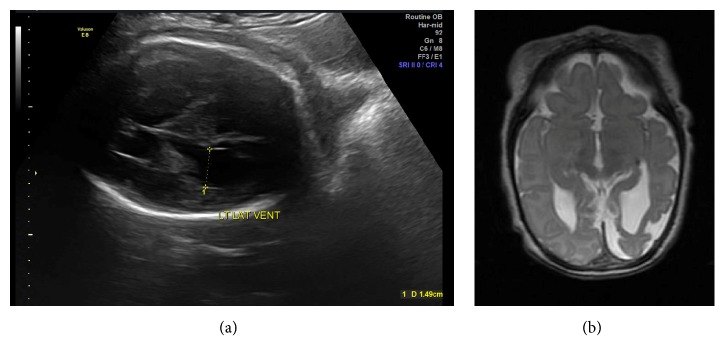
Fetal intracranial imaging and postnatal follow-up. (a) Ultrasound at 35-week gestation demonstrates unilateral ventriculomegaly. (b) MRI at day 4 of life shows cystic encephalomalacia of the bilateral frontal and left parietal lobes and artifact consistent with a previous intraventricular hemorrhage, hemorrhagic contusion, and subdural hematoma.
